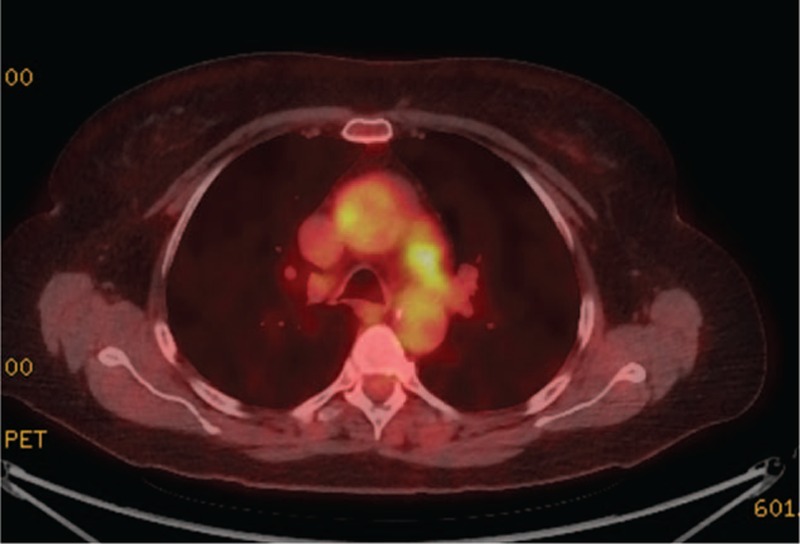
Post-rituximab PET imaging showing decreased FDG avidity of the ascending thoracic aortic wall and soft tissue mass encasing the left pulmonary artery (comparison: Fig. 5B).
